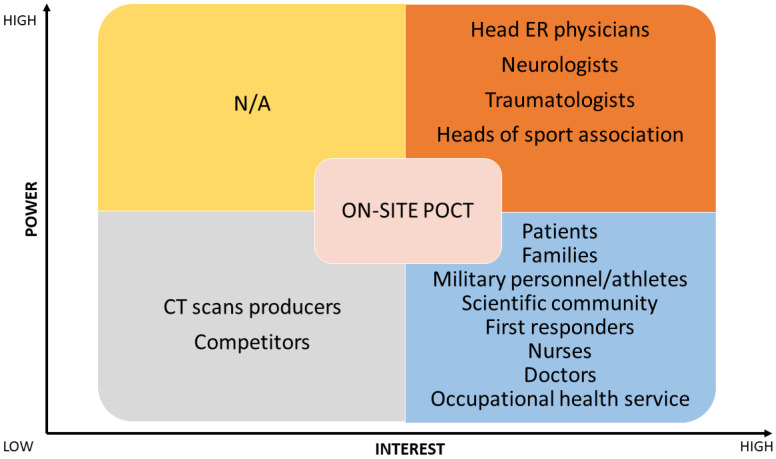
Stakeholder matrix for introducing testing as a point-of-care testing (POCT) on-site. ER, emergency room; CT, computed tomography.
